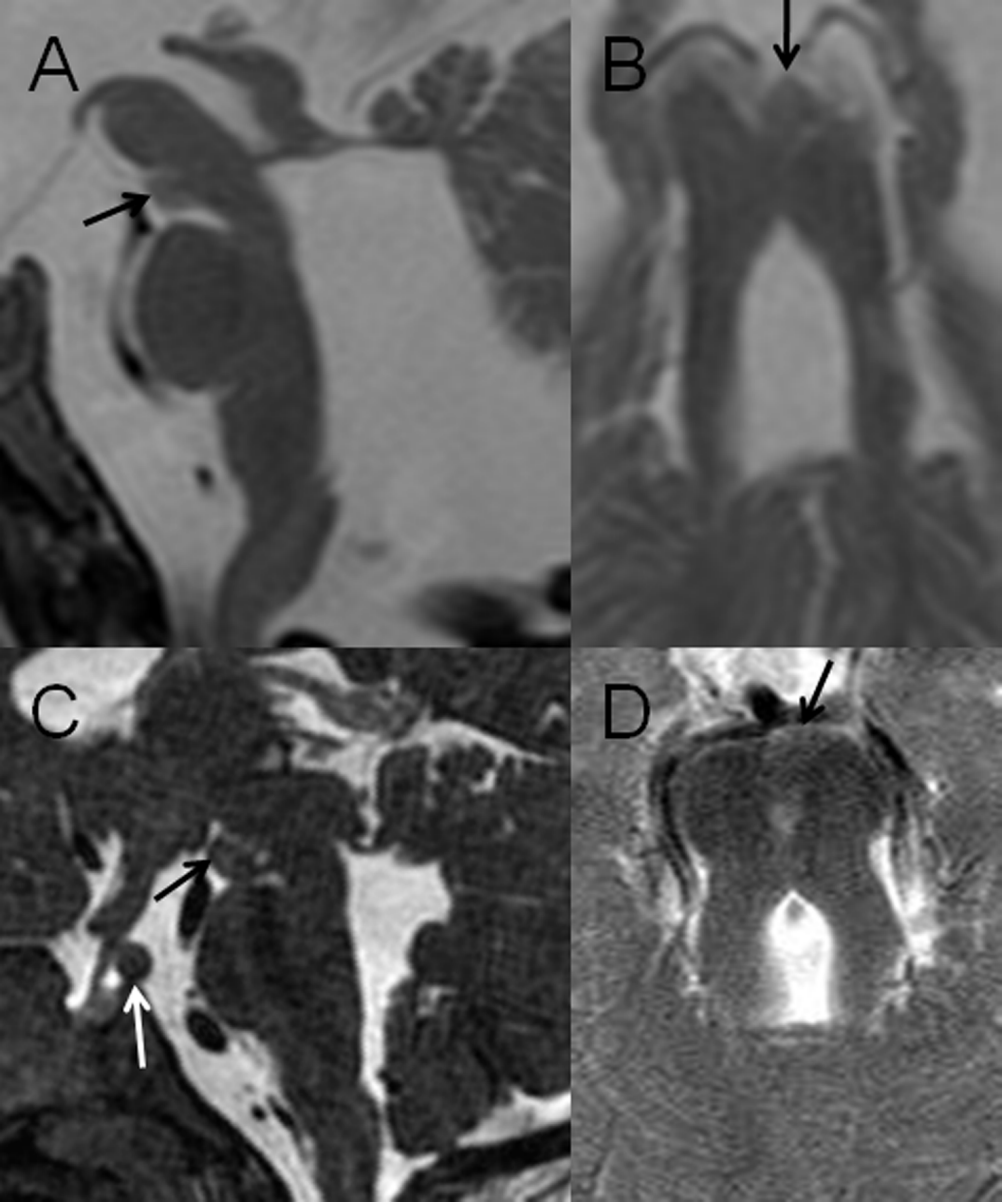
A, midsagittal and B, axial T2-weighted MR images of a 5-month-old infant with OFD VI, and C, midsagittal constructive interference in steady-state (CISS) and D, axial T2-weighted MR images of a 2-year old child with OFD VI. In both patients there is a grey matter isointense, nodular tissue mass in the interpeduncular fossa representing an interpeduncular heterotopia (black arrows). Additionally, C demonstrates a hypothalamic hamartoma (white arrow) and a horizontal septum at the distal end of the Sylvian aqueduct is noted on A.
